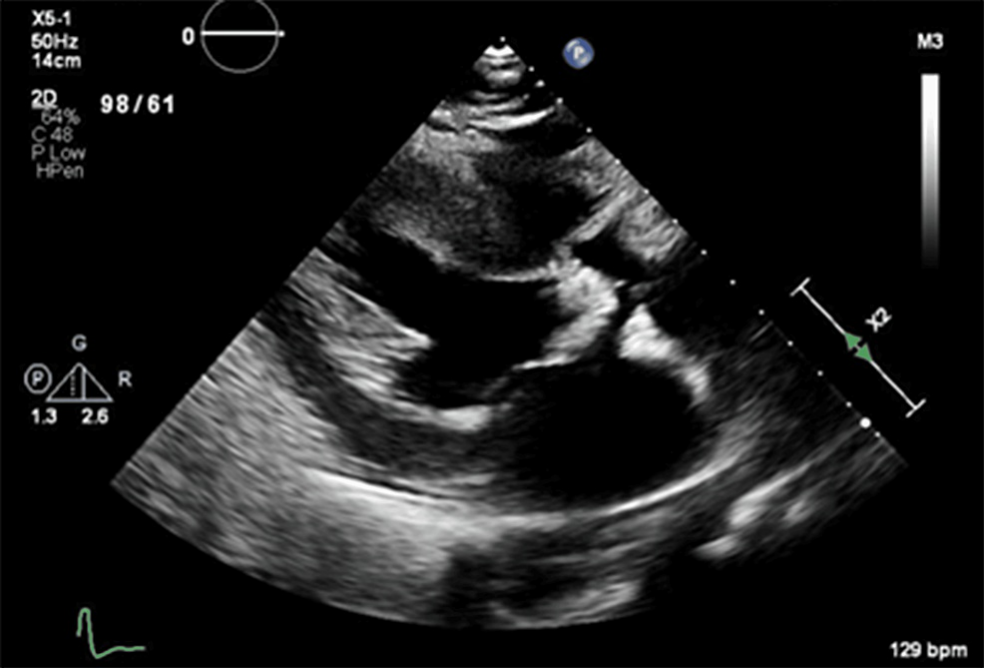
Parasternal long-axis view on transthoracic echocardiography demonstrating severe calcification of the aortic valve
